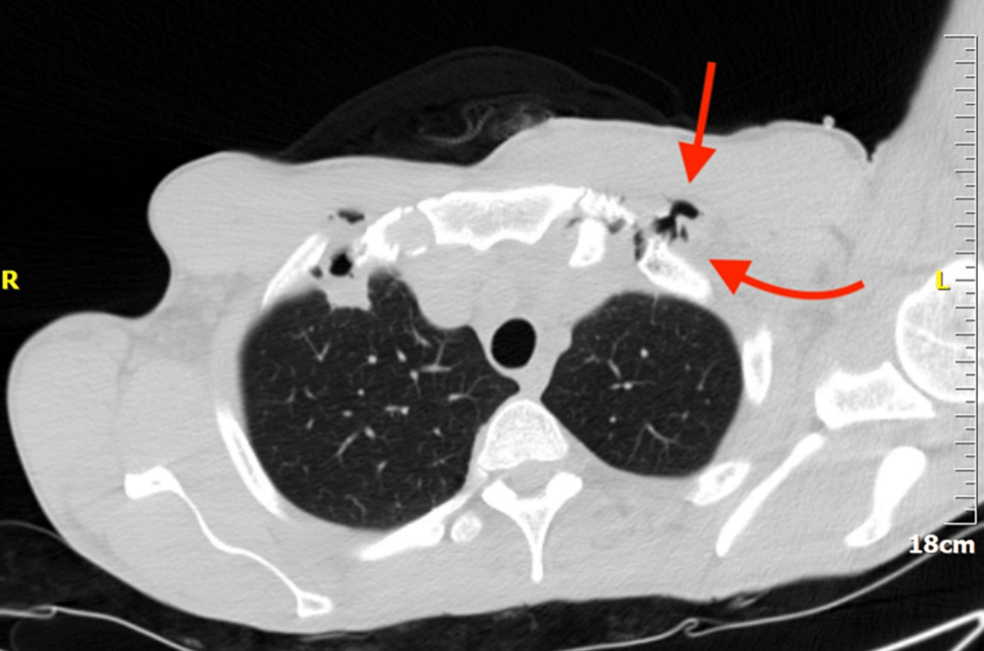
Chest CT scan, lung window. CT: computed tomography
